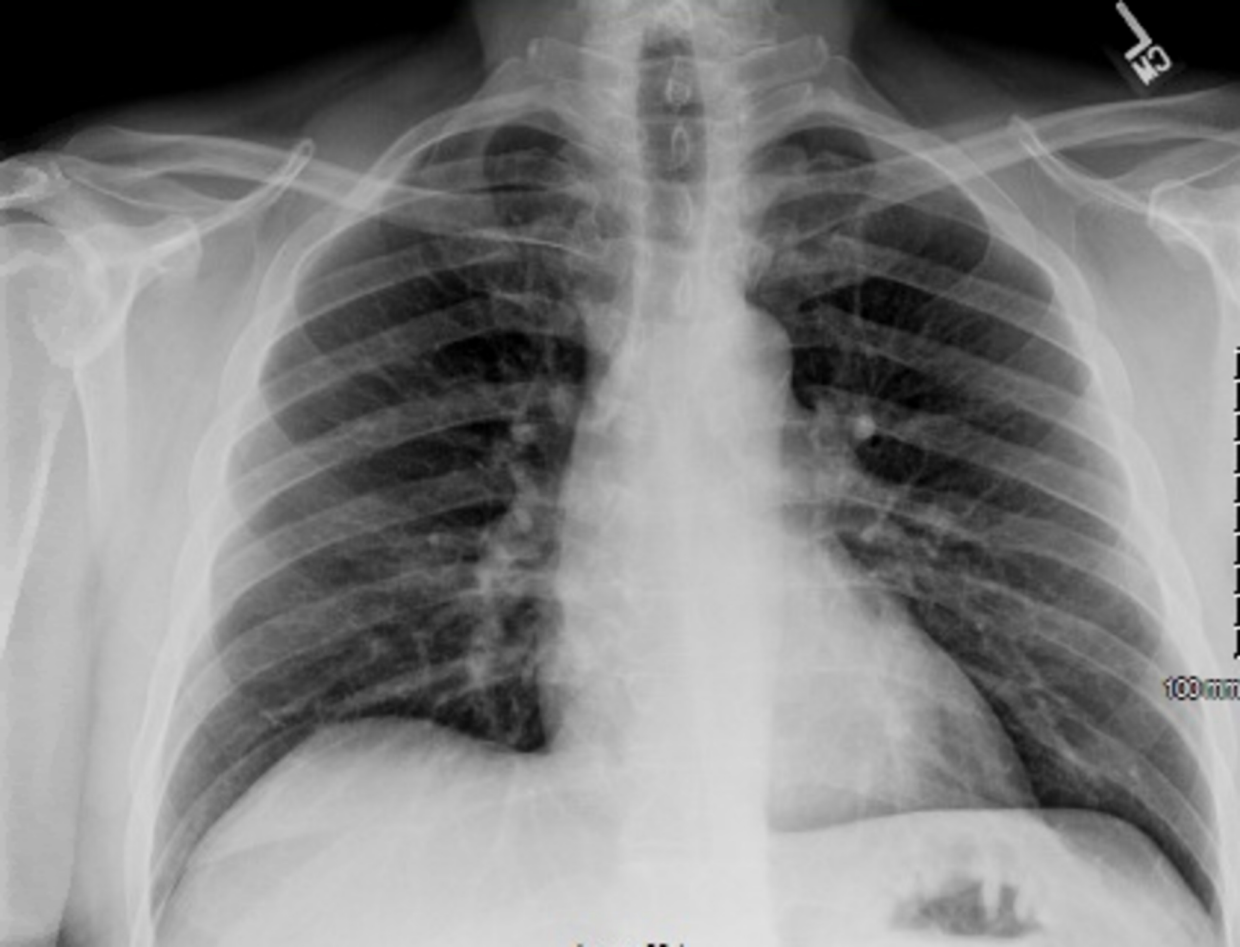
Chest X-ray did not show any acute abnormality.
